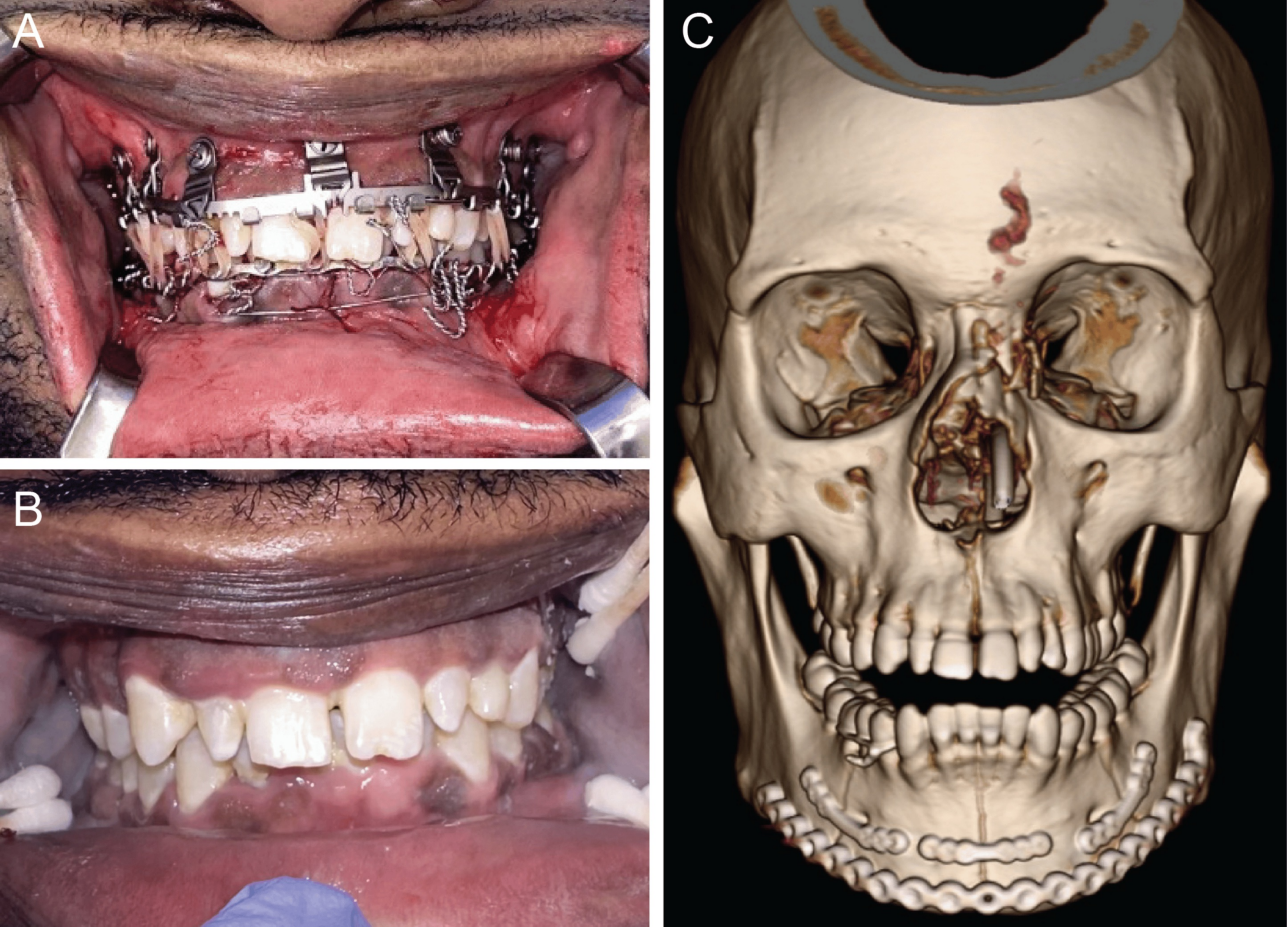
Postoperative occlusion with intermaxillary fixation, 3D reconstruction of maxillofacial computed tomography scan, and occlusion at two-week follow-up examination. (A) Clinical photograph showing intermaxillary fixation with 26-gauge wires around hybrid arch bars. (B) Clinical photograph of patient in occlusion following removal of intermaxillary fixation. (C) 3D reconstruction of full-face computer tomography scan after removal of intermaxillary fixation showing successful reduction and fixation of the mandibular fracture sites
